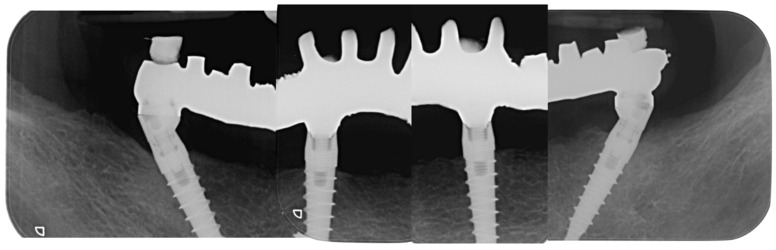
Implant X-rays immediately after load. (March 2022).
